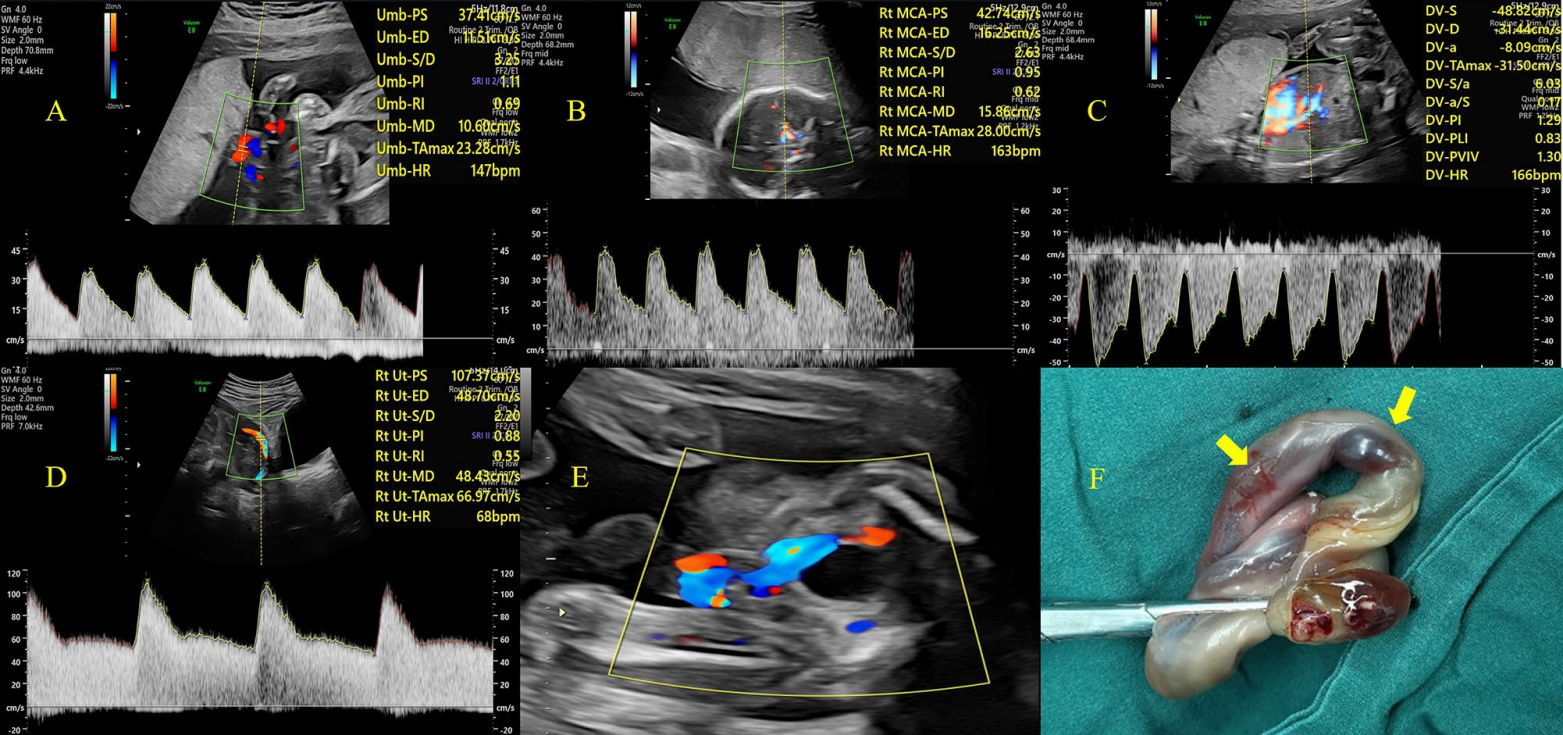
Hemodynamics arrangement of the UAT fetuses with stillbirth (Case 2 in Table 3). A Waveforms from UA, Doppler parameters were both within normal range. B Waveforms from MCA, the value of PI was abnormal (<5th centile for gestational age). C Ductus venosus Doppler recording showed the ‘a-wave’, the value of PIV was abnormal (> 95th centile for gestational age). D Waveforms from UtA, Doppler parameters were both within normal range. E Colour Doppler flow showed only one umbilical artery in the section of bladder. F Section through the umbilical cord: thromboembolism in one UA, dark brown appearance (yellow arrow)
